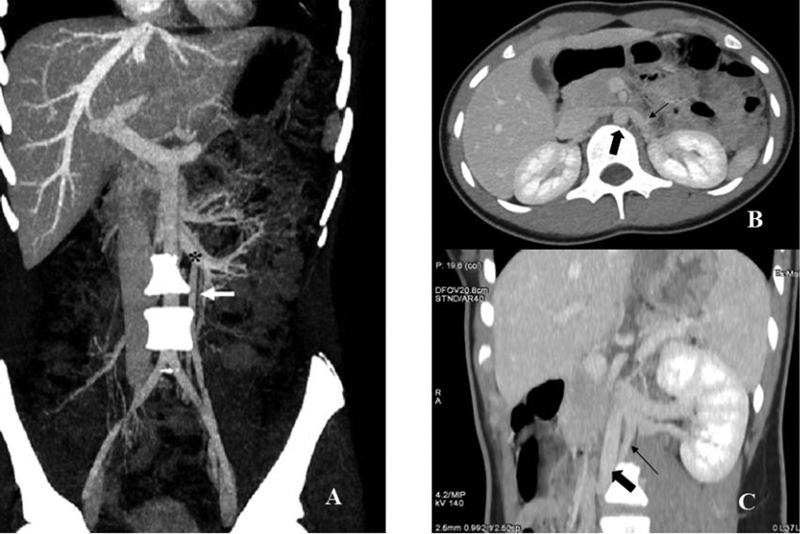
Abdominal pelvic computed tomography scan with contrast. (
